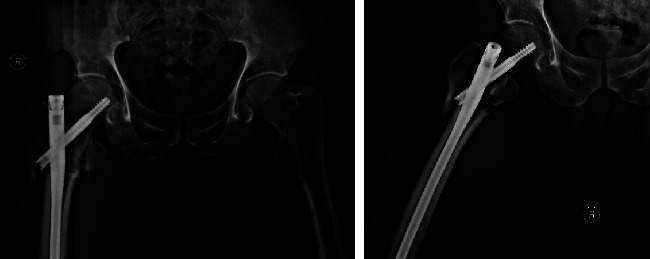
Postoperative X-ray of a patient with intertrochanteric fracture of femur.
